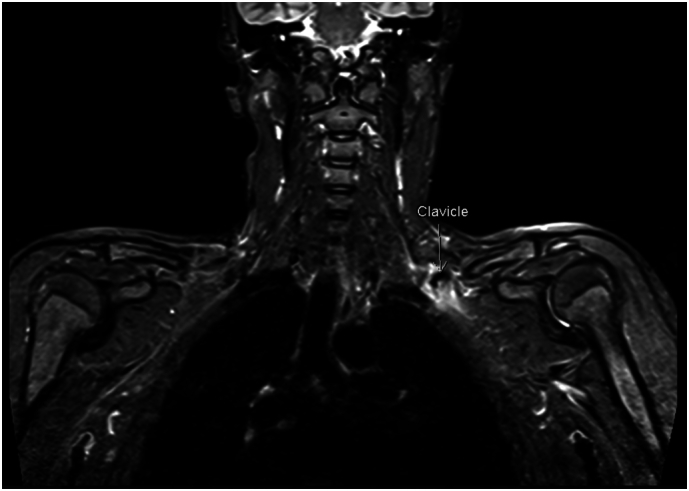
MRI Brachial plexus showing thickened and oedematous brachial plexus, at the site of clavicle fracture impingement.
